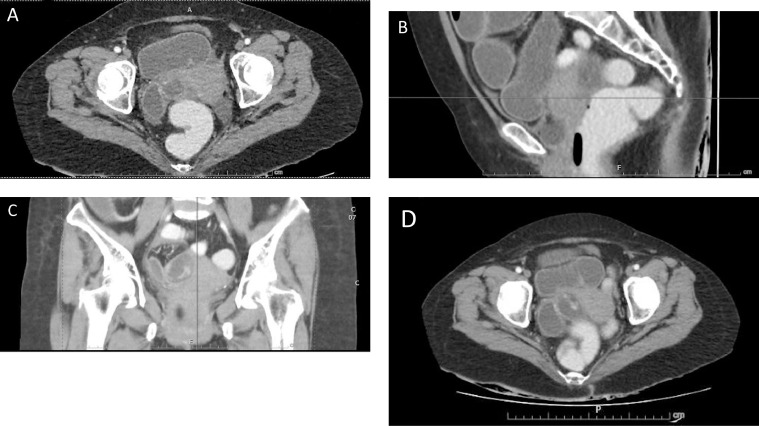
CT of the abdomen at axial (A), coronal (B) and sagittal (C) view showed a short segment of narrowing at the distal ileum (17 cm from ileocecal junction) positioned immediately inferior to the right fallopian tube.
